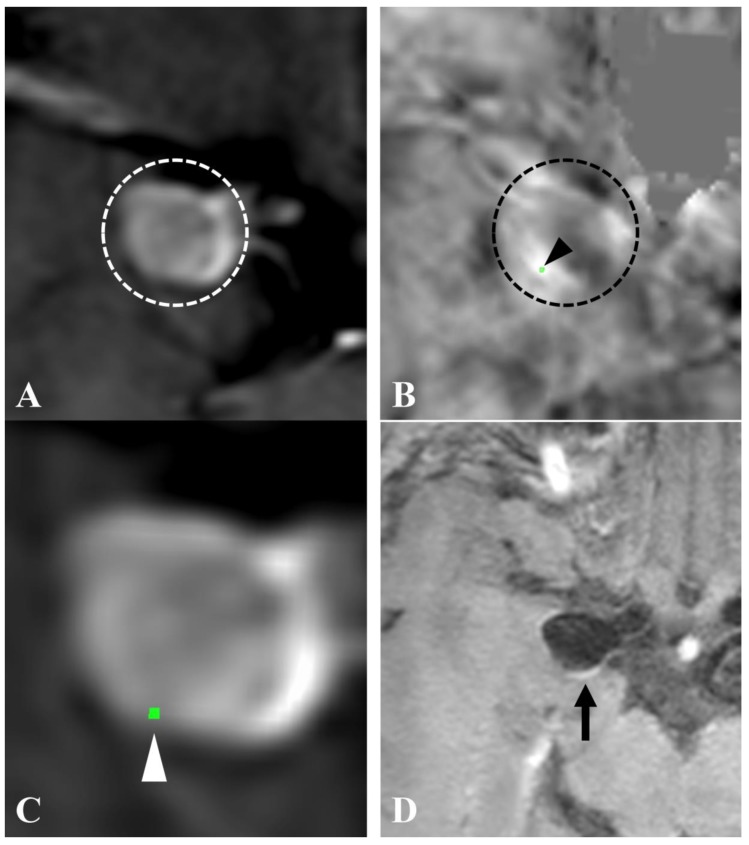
QSM and vessel wall imaging (VWI) of an intracranial aneurysm with SH. A subject presented to our neurosurgical clinic with sudden onset of a worst headache. There was no evidence of subarachnoid hemorrhage (SAH) in head CT and lumbar puncture. A right internal carotid artery–posterior communicating artery aneurysm was described in time-of-flight MR angiography (TOF-MRA) ((A), circle) and QSM ((B), circle). An area of high susceptibility, which was based on the threshold of 0.1 parts per million, was revealed ((B), arrowhead). The QSM image overlapped on TOF-MRA (C) indicated the area of high susceptibility at the interface of the aneurysmal wall (arrowhead). Postcontrast VWI (D) showed AWE in the aneurysmal wall (arrow).
